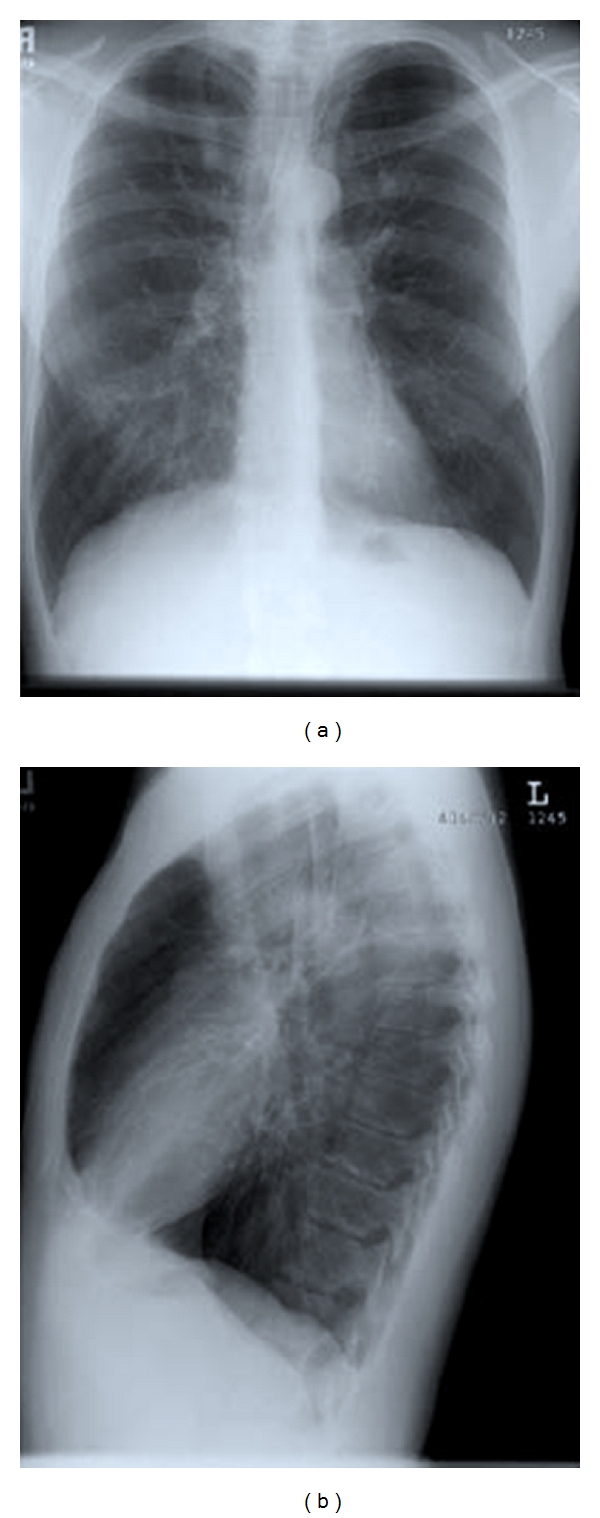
Chest roentgenogram at 35 years of age shows hyperinflation and increased linear markings particularly in the right lower lung zones and perihilar regions corresponding to a Brasfield et al. score of 15 [6].
